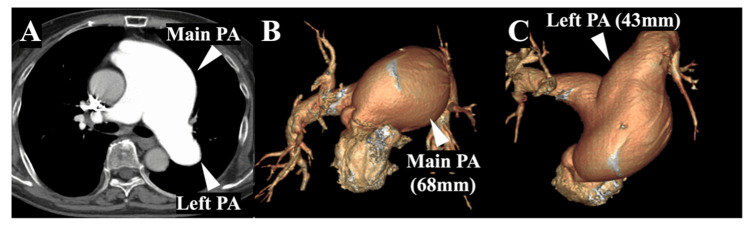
Preoperative CT Contrast-enhanced CT revealed a massively dilated main PA (68 mm) and proximal left PA (43 mm) (A). The PA is shown on 3D CT (B, C). PA: pulmonary artery
